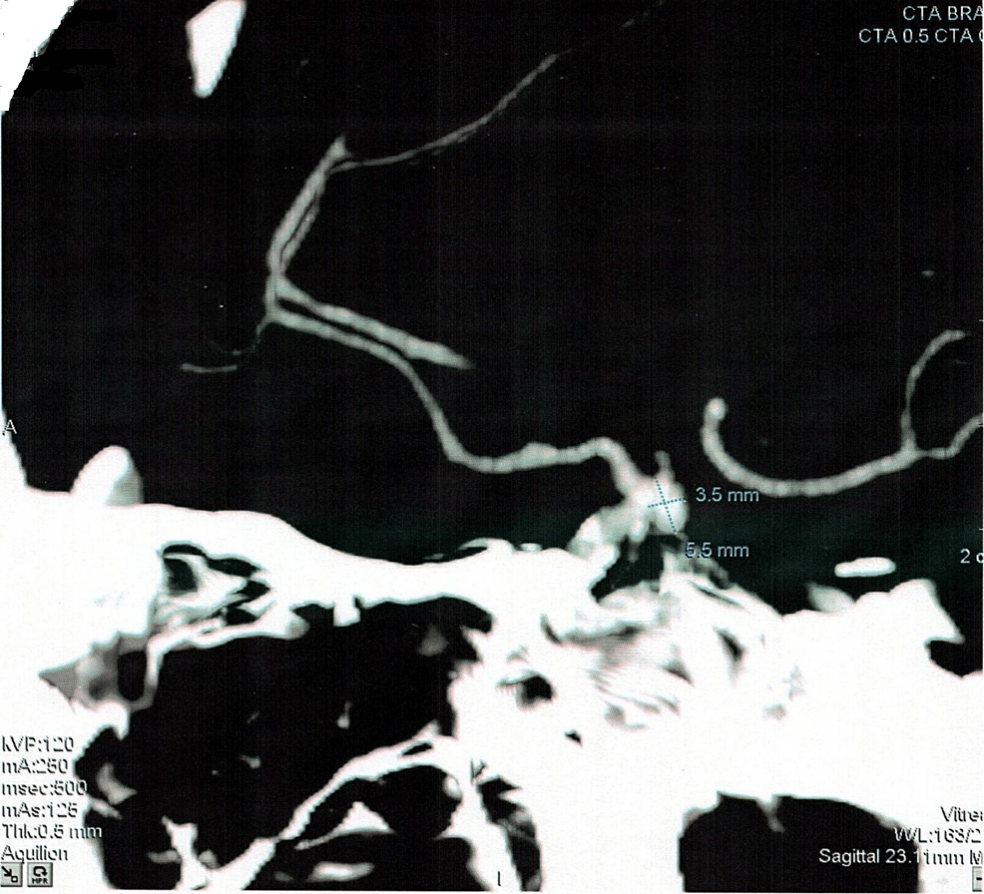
Computed tomography angiogram depicting the size of the aneurysm
